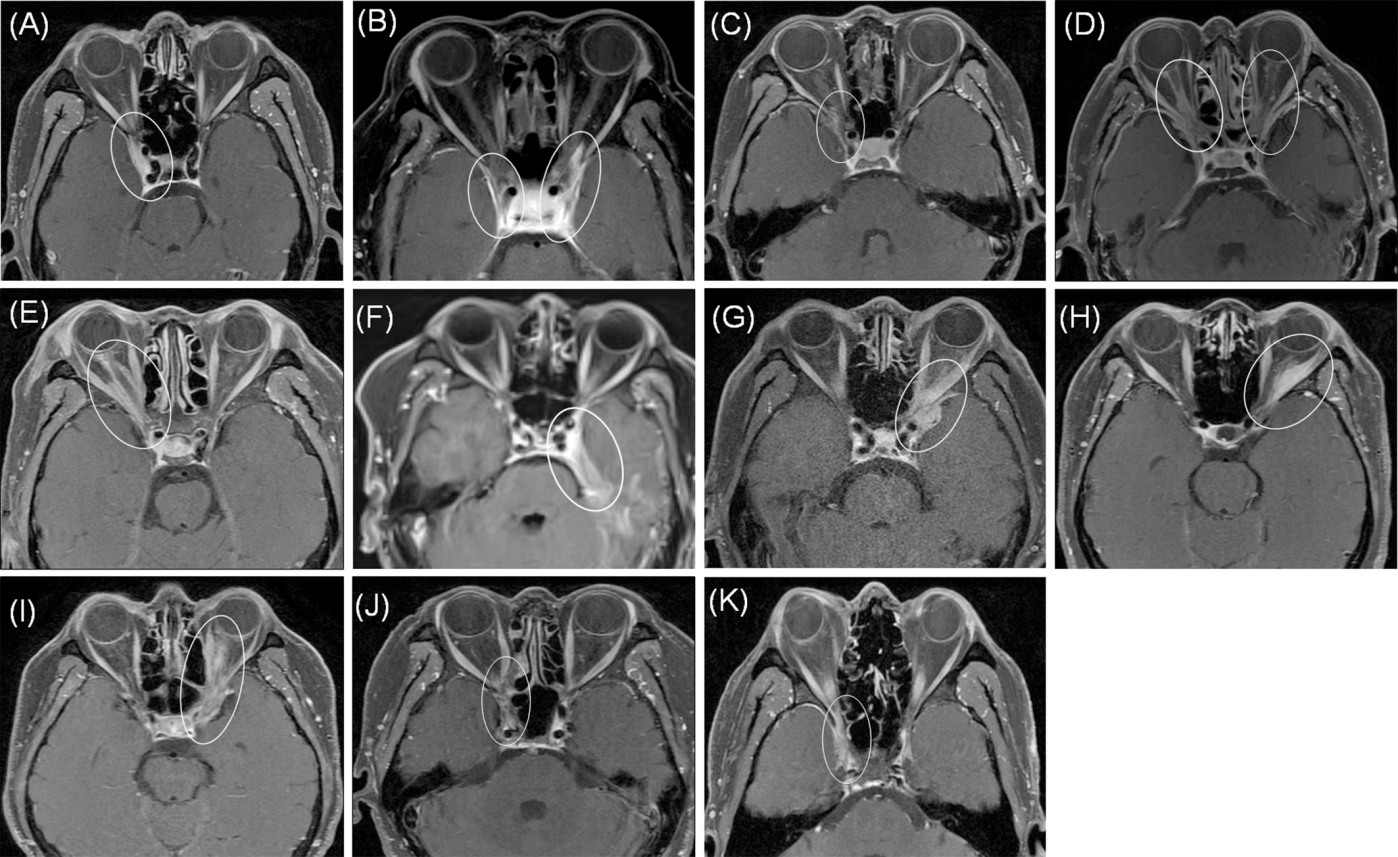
MRI axial showed abnormal enhancement of lesions (circle) from patient 1 to 11 (A–K). Patients 4, 5 and 9 (D, E, I) showed enhancement of the optic nerve sheath. All patients showed involvement of cavernous sinus and dura.
